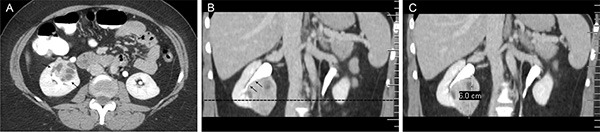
An example of PADUA radiological anatomical features analysis According to the PADUA scoring system, the following radiological anatomical features are evaluated in an axial contrast-enhanced CT image (A) and coronal multiplanar reformatted contrast-enhanced CT image (B and C). 1) Exophytic rate: a tumor that is < 50% exophytic is graded as 2 points (A and B); 2) Longitudinal location: a tumor crossing the sinus line (dotted line) > 50% is graded as 2 points (B); 3) Rim location: a tumor located at the medial rim is graded as 2 points (A and B); 4) Renal sinus: a tumor that involved the renal sinus (arrows in A) is graded as 2 points; 5) UCS: a tumor that involved the UCS (arrows in B) is graded as 2 points; 6) Tumor size: a 6.0 cm tumor is graded as 2 points (C); 7) Face location: a tumor on anterior faces of the kidney is indicated with a letter “a” (Figure A). Thus the total PADUA score of this case is 12a.
